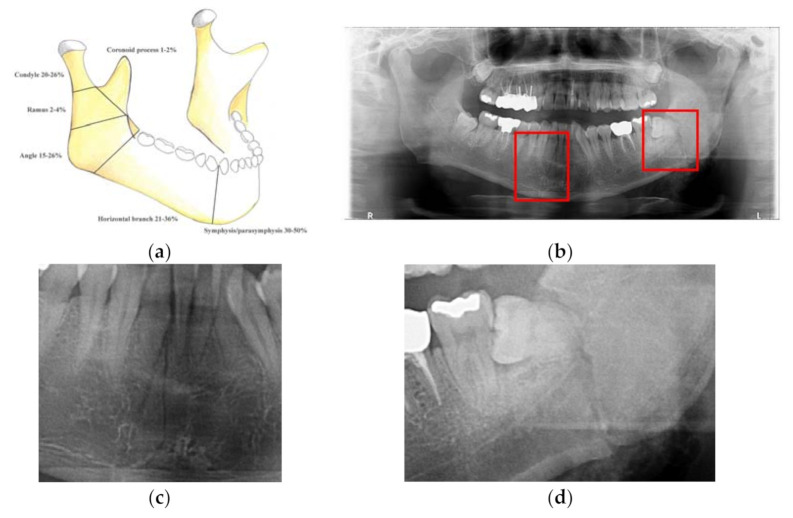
Examples of mandibular fracture: (a) anatomic area of mandibular fracture [2], (b) mandibular fractures (red boxes) in panoramic radiographs, (c) linear fracture on parasymphysis area, and (d) shear fracture on angle area.
